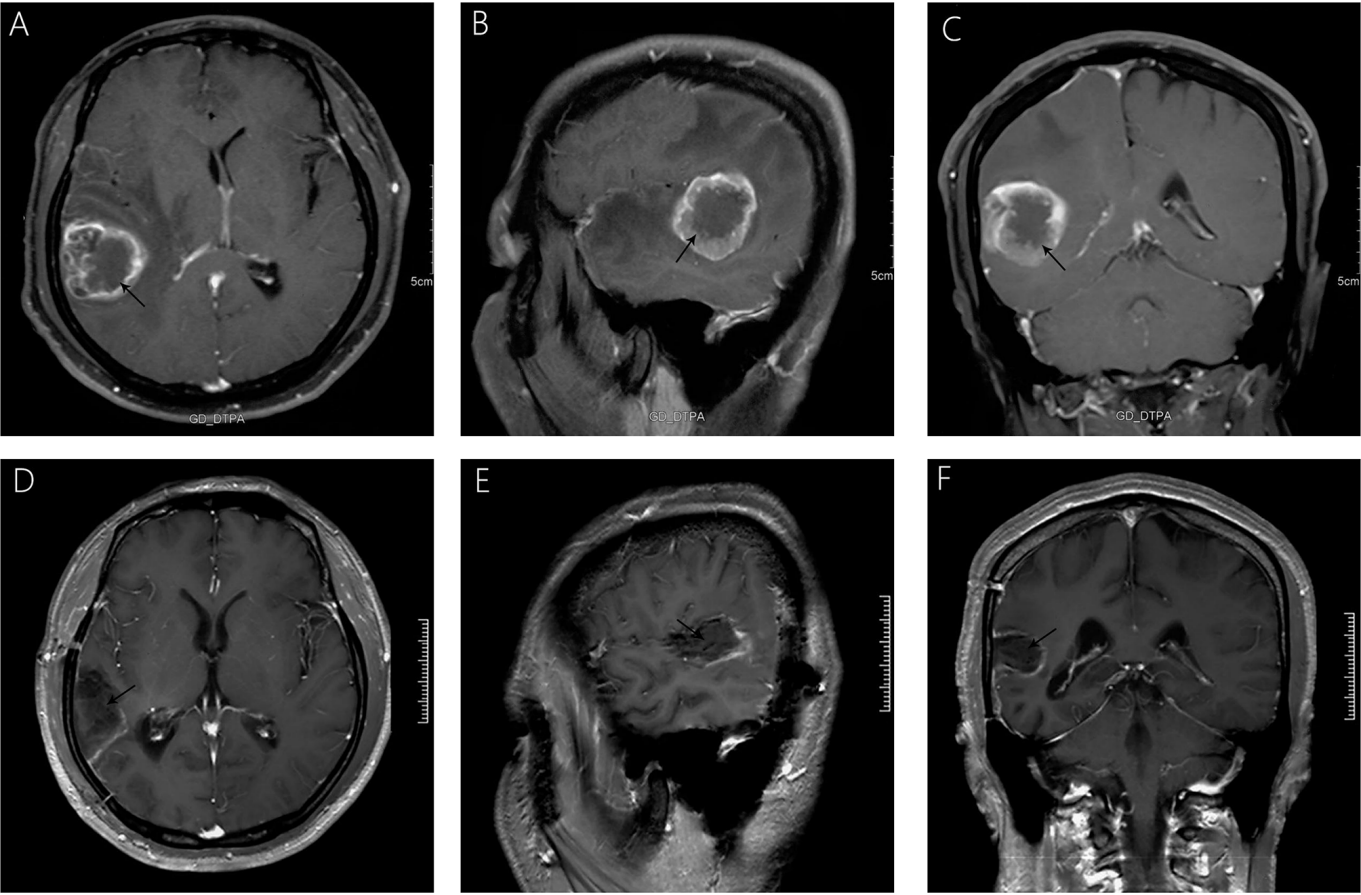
Significant heterogeneous enhancement is observed with evident edema after gadolinium administration (A–C). A follow-up MRI, 3 months after surgery (D–F), showed that the lesion was completely removed, without any signs of recurrence.
